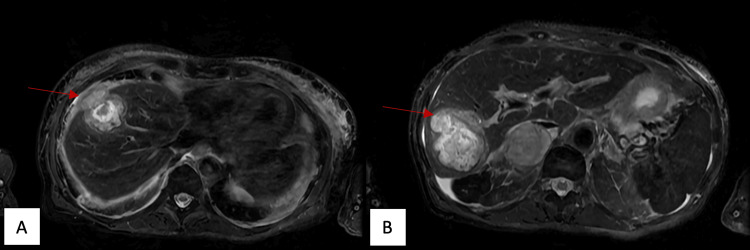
Fat-saturated T2 MRI images showing high internal T2 signal with mildly elevated peripheral T2 signal in the superior (A) and inferior (B) lesions
